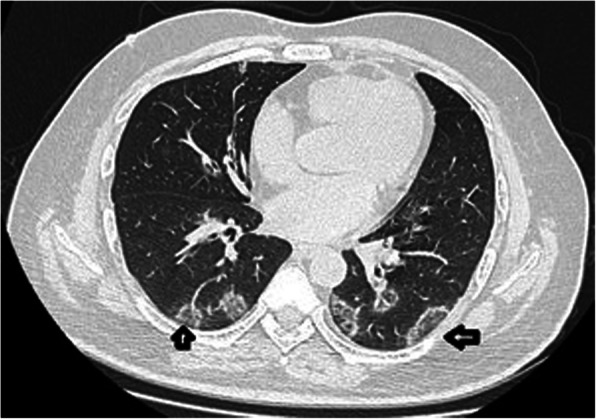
Axial HRCT chest image of the patient (120 KV 100 mAs bone algorithm) at the level of heart showing bilateral multifocal ground glass lesions (open arrows) in both lower lobes in peripheral distribution
